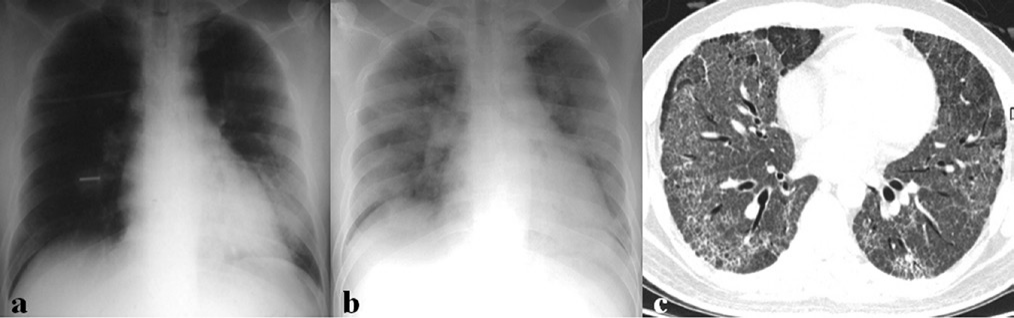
A 31-year-old male with SARS-CoV infection. (a) Initial chest radiograph (CXR) obtained on the day of admission shows unilateral lobar consolidation in left lung. (b) Follow-up CXR obtained 4 days after (a) shows rapid progression with bilateral diffuse ground-glass opacities (GGOs) and consolidation. (c) Follow-up chest CT obtained 40 days after admission shows bilateral diffuse GGOs with superimposed intralobular interstitial thickenings and reticulation, suggesting pulmonary fibrosis.
